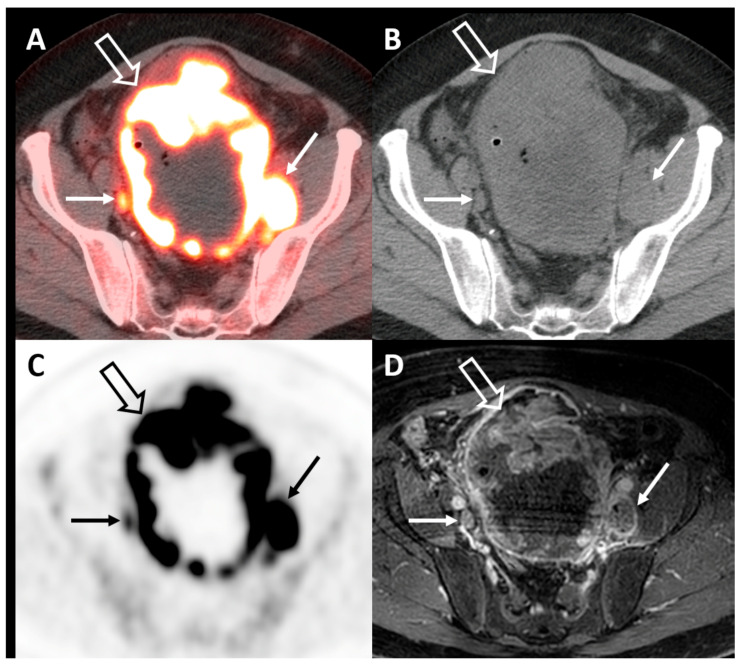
Axial fused FDG-PET/CT (A), CT (B), PET (C), and corresponding T1-fat saturated postcontrast MRI (D) demonstrating an extensive hypermetabolic mass involving nearly all of the bladder wall (open arrow) and multiple pelvic lymph node metastases (closed arrow), some of which measure less than 1 cm in short axis and are more easily identified on PET/CT vs. CT or MRI alone.
